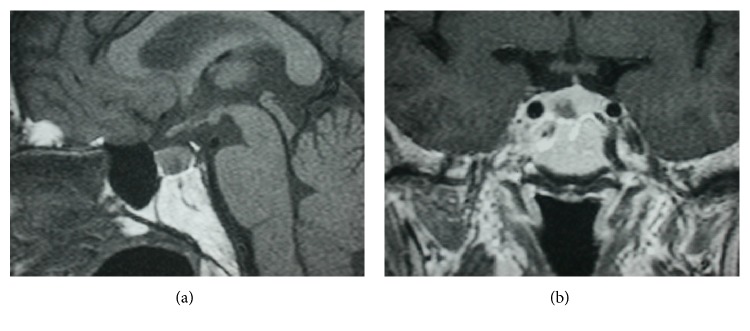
MRI pituitary gland (T1-weighted, sagittal view) showed a 6 mm hypointensity sellar lesion with irregular margin (a). On coronal postcontrast T1-weighted image, the lesion remained hypointensity while the gland showed marked homogenous enhancement (b).
